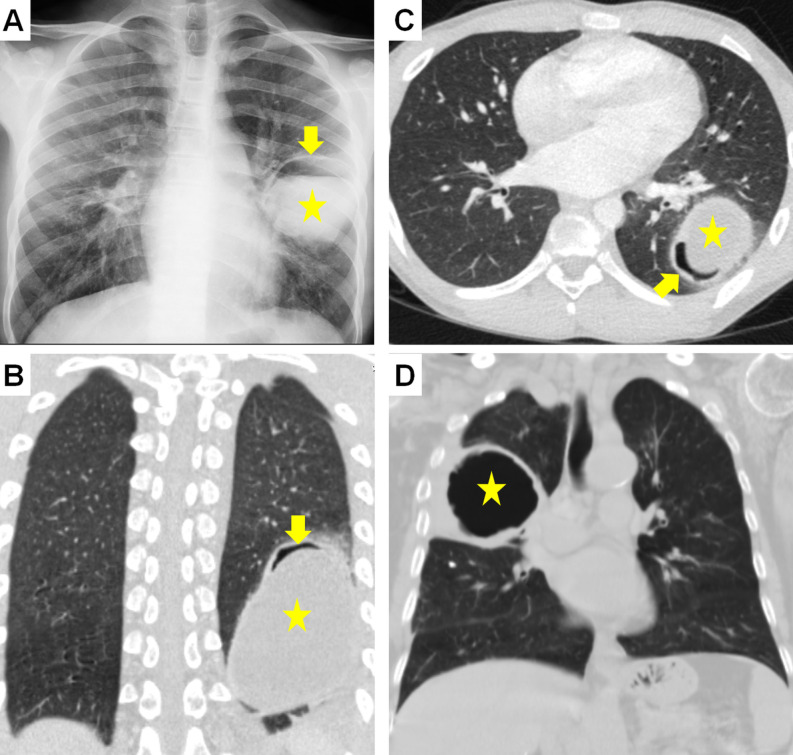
Radiological signs of contained cyst rupture and cyst emptying. In different cases, chest radiograph (A) and coronal parenchymal computed tomography (CT) (B) show the “air crescent or meniscus or moon sign” formed by air entering between the pericyst and laminar membrane (arrow), although the hydatid cyst (HC) is not yet ruptured (asterisks). Axial CT (C) shows the “inverse air crescent sign” (arrow) formed by dissection from the posterior of the HC membranes (asterisk). Coronal CT (D) shows the “empty or dry cyst sign” (asterisk) consisting only of the pericyst wall remaining after the cyst content is completely emptied.
